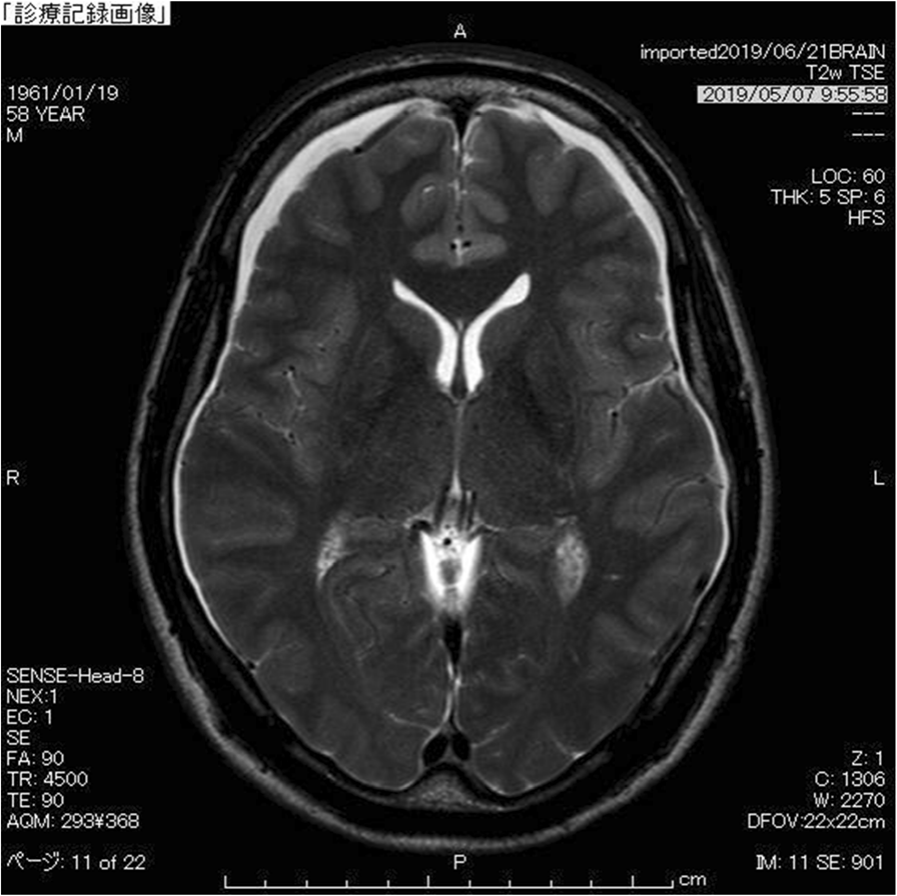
Subdural effusion at the onse. Bilateral subdural effusion was demonstrated by T2-weighted image of brain magnetic resonance imaging (MRI)
